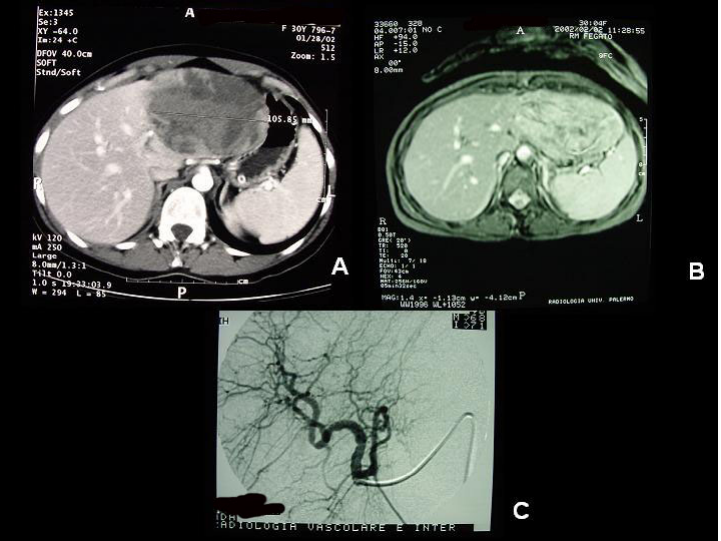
A: Neoformation in the left lobe (CT imaging) B: Neofomation in the left lobe (MR imaging)) C: Angiography: no vision of the left hepatic lobe or neoformation.
